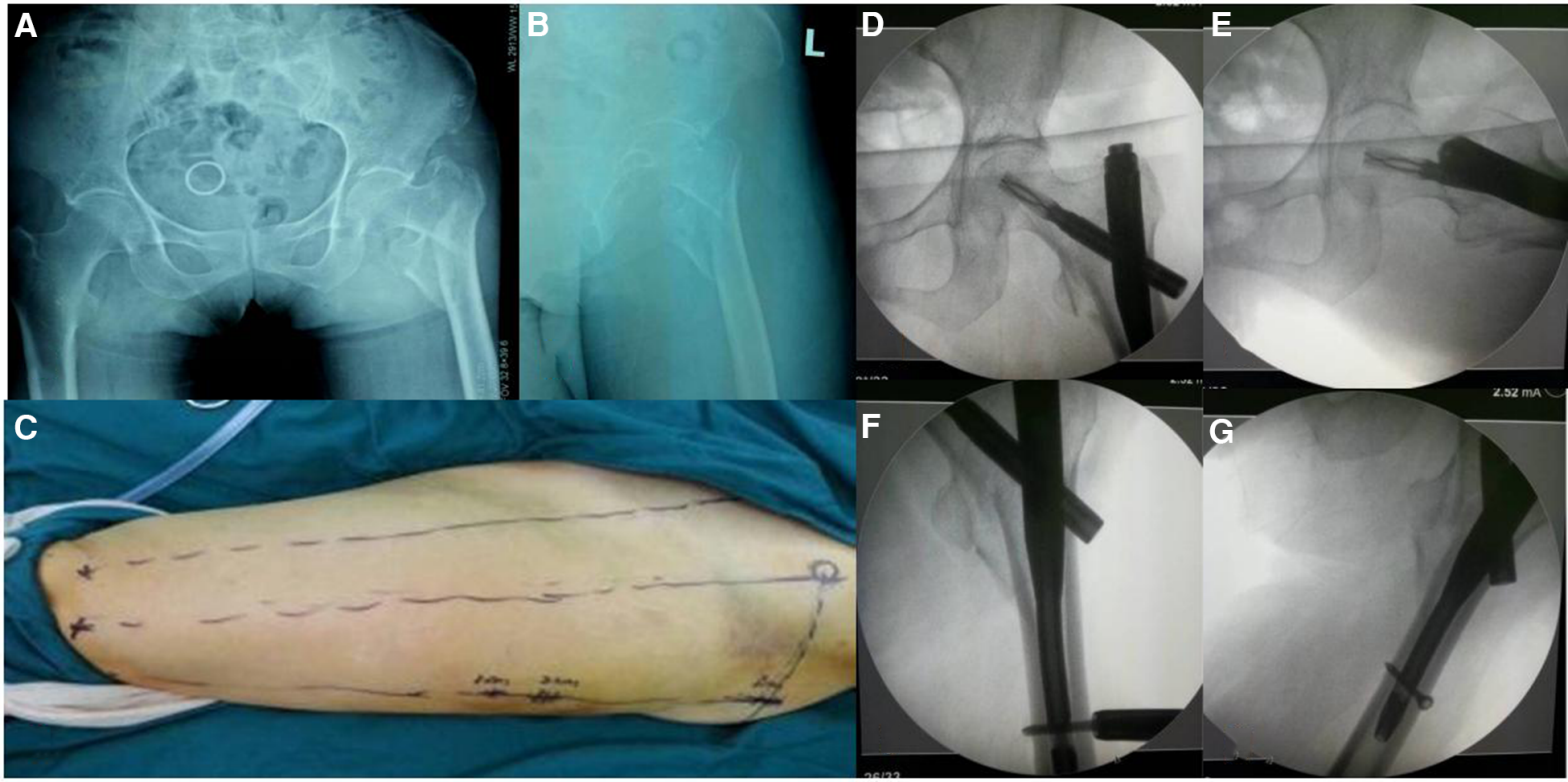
Preoperative anterolateral x-rays of the patient's hip joint (A,B) preoperative localization of skin incision was performed by the “3-2-1” body surface localization method (C) postoperative anterolateral x-rays of the patient's hip joint showed good fracture reduction (D–G).
